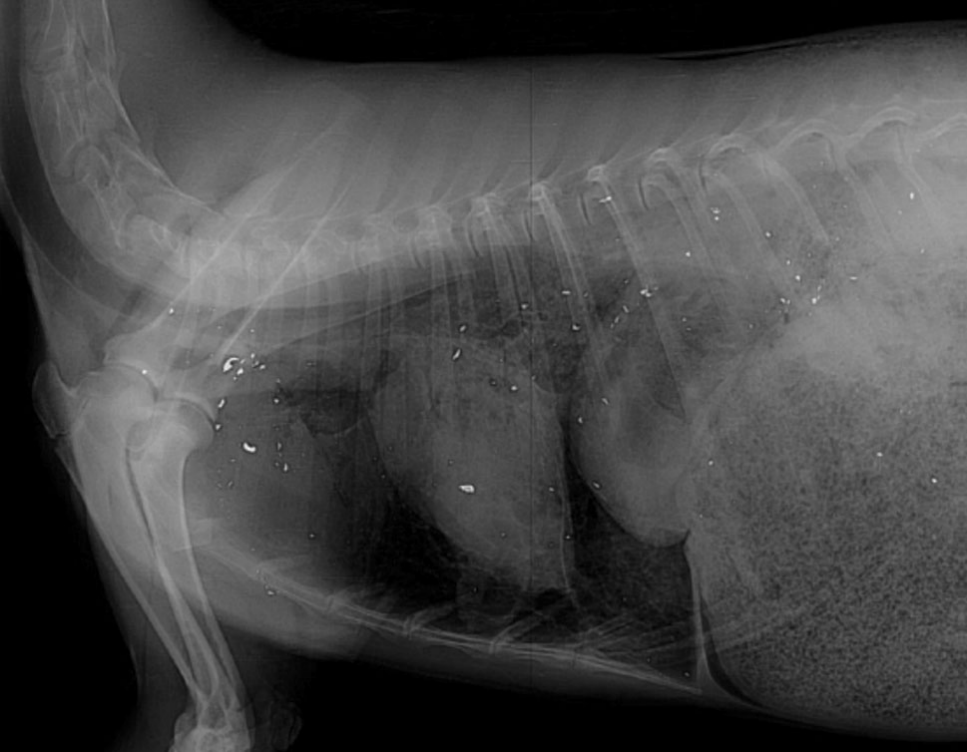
Radiograph of a roe deer shot with a single unbonded lead rifle bullet, showing the extent of the bullet’s fragmentation and the distance of fragments’ spread from the entry site. Most of the small fragments would not likely be removed prior to butchering and retail sale, thereby exposing the consumer. Photo credit, Oliver Krone, Leibniz Institute for Zoo and Wildlife Research, Berlin, Germany
